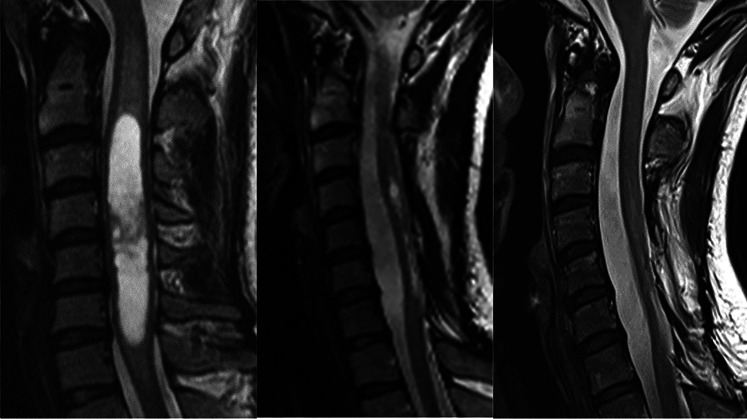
Cystic ependymoma. T2 weighted MRI preoperatively, 1 year and 7 years postoperatively
